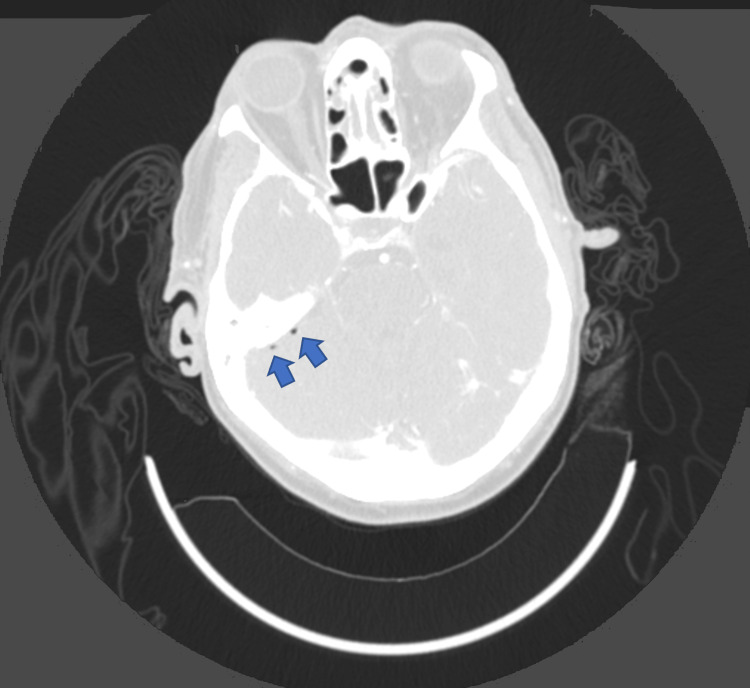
Computed tomography angiography of the head in the axial view demonstrating pneumocephalus along the right tentorium (blue arrows).
